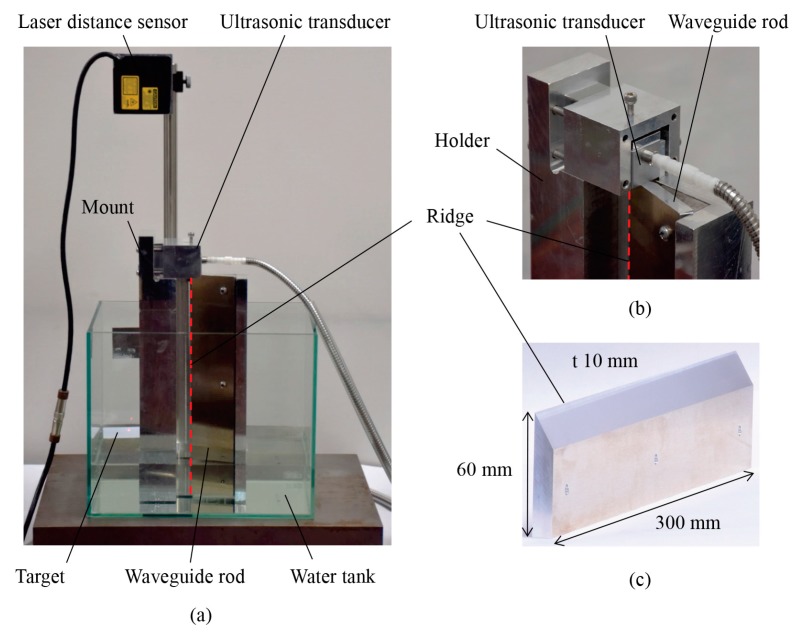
(a) Picture of experimental setup for measuring liquid-level. (b) Contact part of ultrasonic transducer and waveguide rod. (c) Waveguide rod. Red dashed line indicates ridge of waveguide rod.
